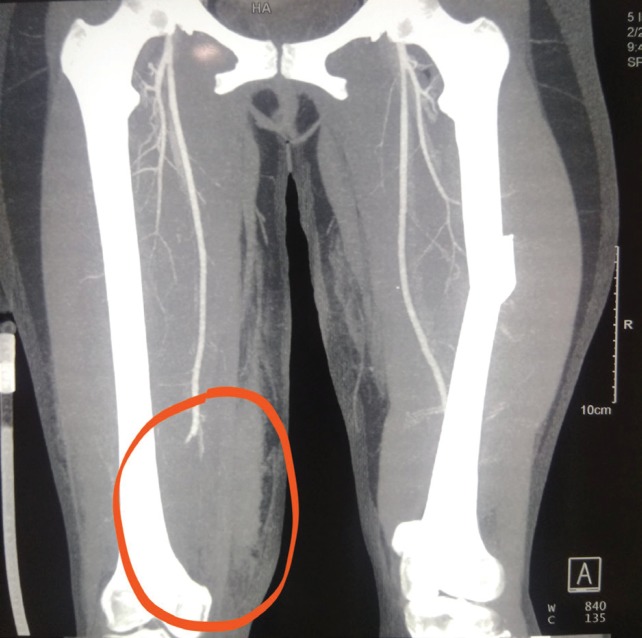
CT angiogram showing non-opacification of right distal superficial femoral artery (encircled)
